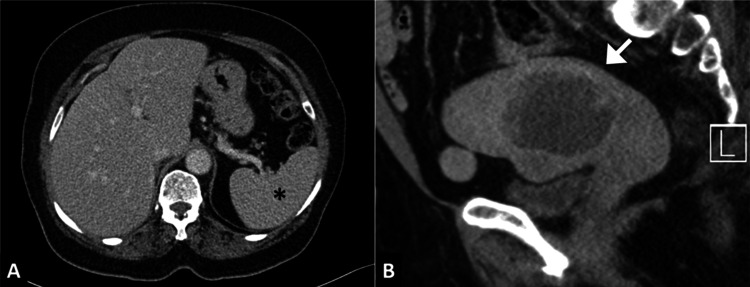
Cross-sectional imaging at initial diagnosis A. Axial contrast-enhanced CT image of the upper abdomen showing a normal-appearing spleen (asterisk).
